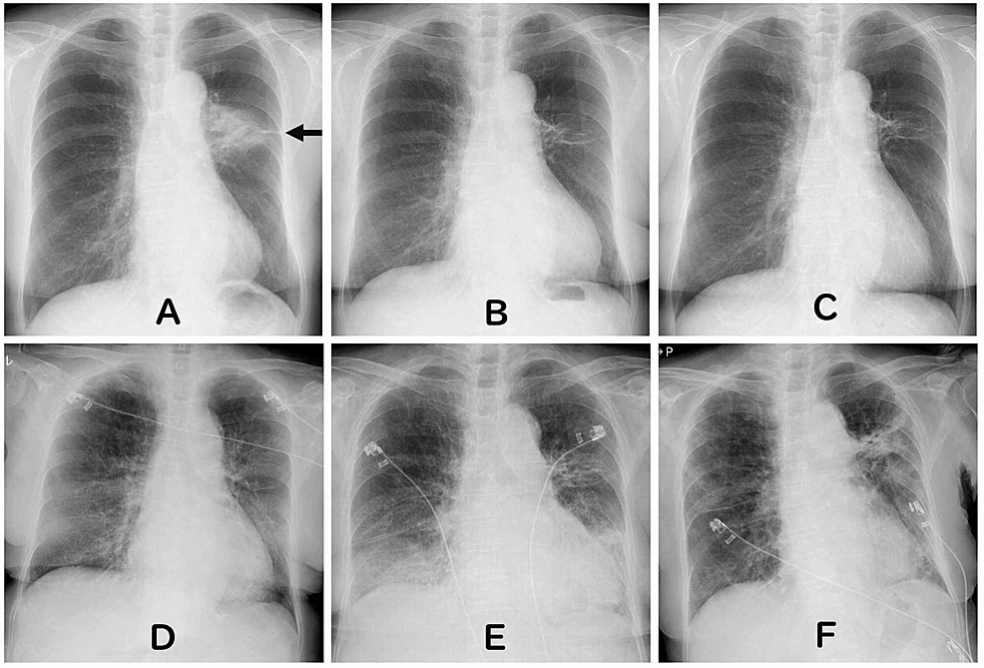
X-p image. (A) X-p five months after curative radiation therapy: radiation pneumonia consistent with the irradiation field was observed. (B) X-p 3.5 years after the curative radiation therapy. This is two weeks before SARS-CoV-2 positivity: radiation-induced pulmonary fibrosis was observed. (C) X-p one day prior to SARS-CoV-2 positivity: no change from two weeks prior. (D) X-p on the day of rapid deterioration of respiratory condition observation (day seven): appearance of ground-glass shadow showing dilated bronchi inside. (E) X-p two days later: a shadow consistent with the irradiation was observed (day nine). (F) X-p 46 days after SARS-CoV-2 positivity. X-p, chest radiograph; SARS-CoV-2, severe acute respiratory syndrome coronavirus 2
